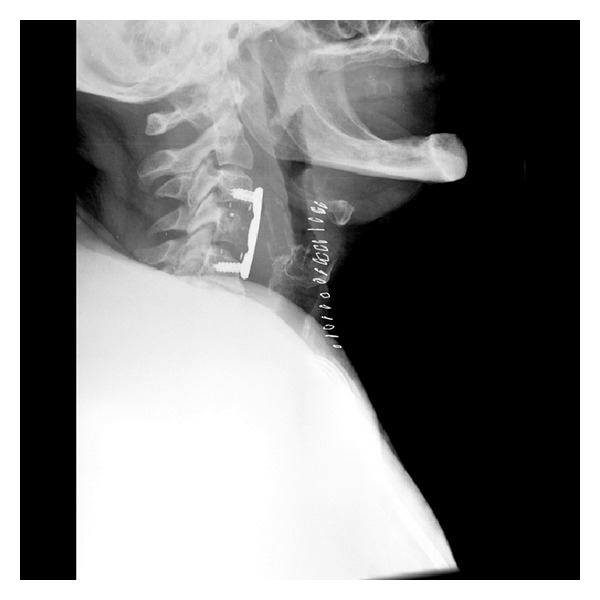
Postoperative lateral radiograph of the cervical spine showing a stable anterior C3–C5 stabilization using a cage and an anterior plate.
